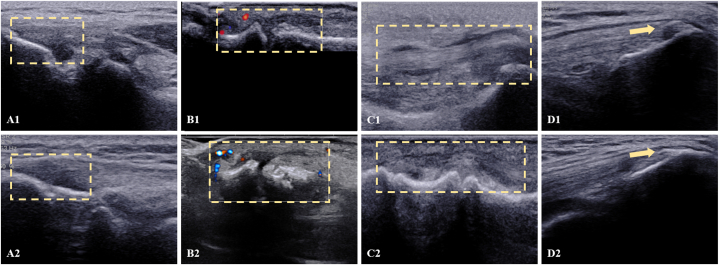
“Difference in US abnormalities between the PsA and Sub-PsA groups.” (A) Fig. 2 PsA (A1-D1) and Subclinical PsA (A2-D2) ultrasound abnormalities. A1, A2: Enthesitis (dotted boxes), manifested as hypoechoic thickened insertion of the enthesis close to the bone; B1, B2: Synovitis (dotted lines), manifested as hypoechoic synovial hypertrophy; C1, C2:Tenosynovitis (dotted boxes), manifested as abnormal hypoechoic tendon sheath widening; and D1, D2: New bone formation (arrows), manifested as hyperechoic bony prominence distant from the bone margin.
